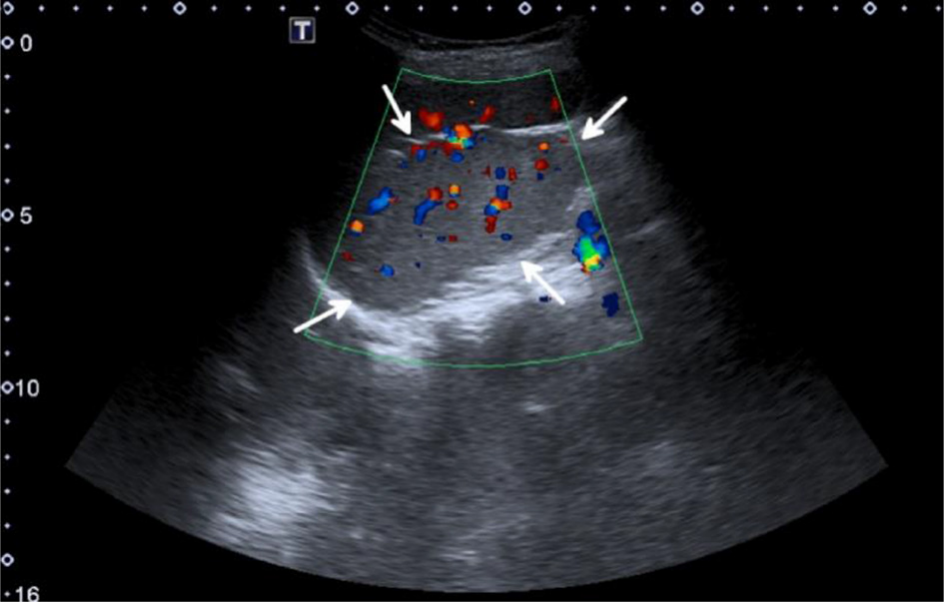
Long axis colour Doppler ultrasonography image of the spleen. The medial (internal) lobe of the spleen (white arrows) seen as a smooth ill-defined bulge from the splenic hilum. The medial splenic lobe demonstrated enriched vascularity like the rest of splenic parenchyma.
